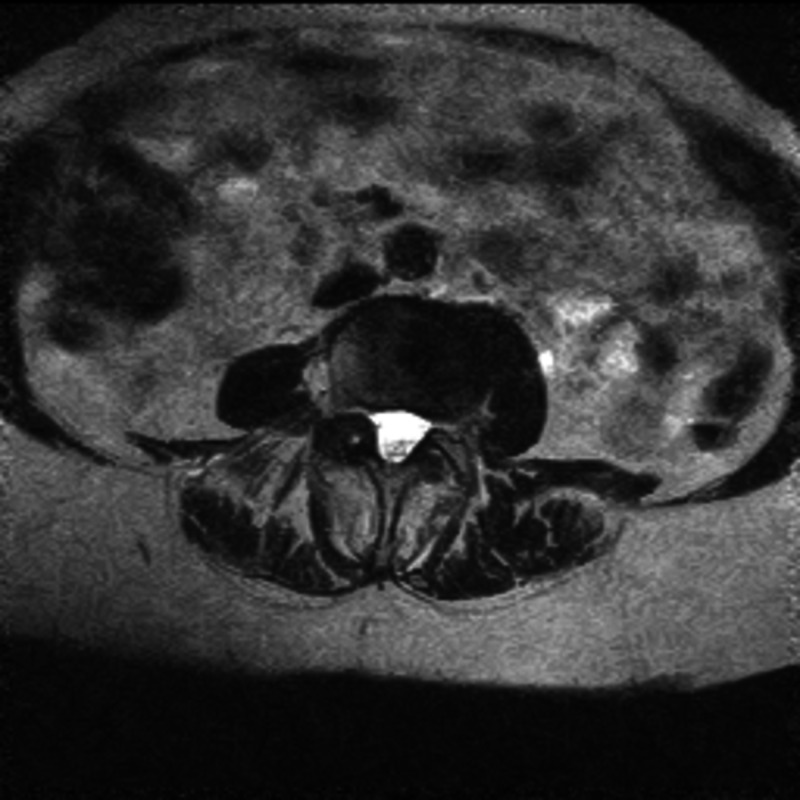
Axial T2 weighted MRI image with negative nerve root sedimentation sign.
